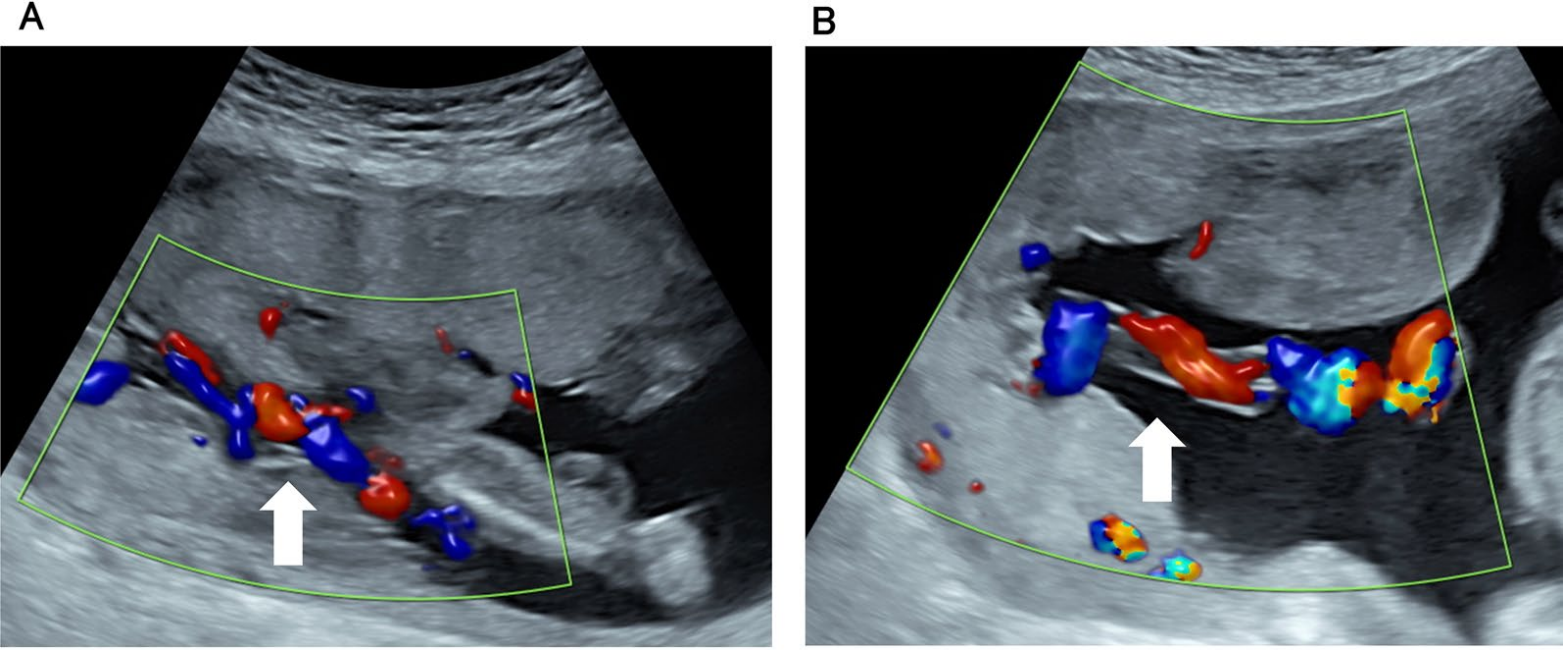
Fetal ultrasonography findings in case 2. A The “sandwich sign,” that is, the umbilical cord sandwiched between the placenta and the fetus, is observed (white arrow). B The umbilical cord compression has resolved (white arrow)
